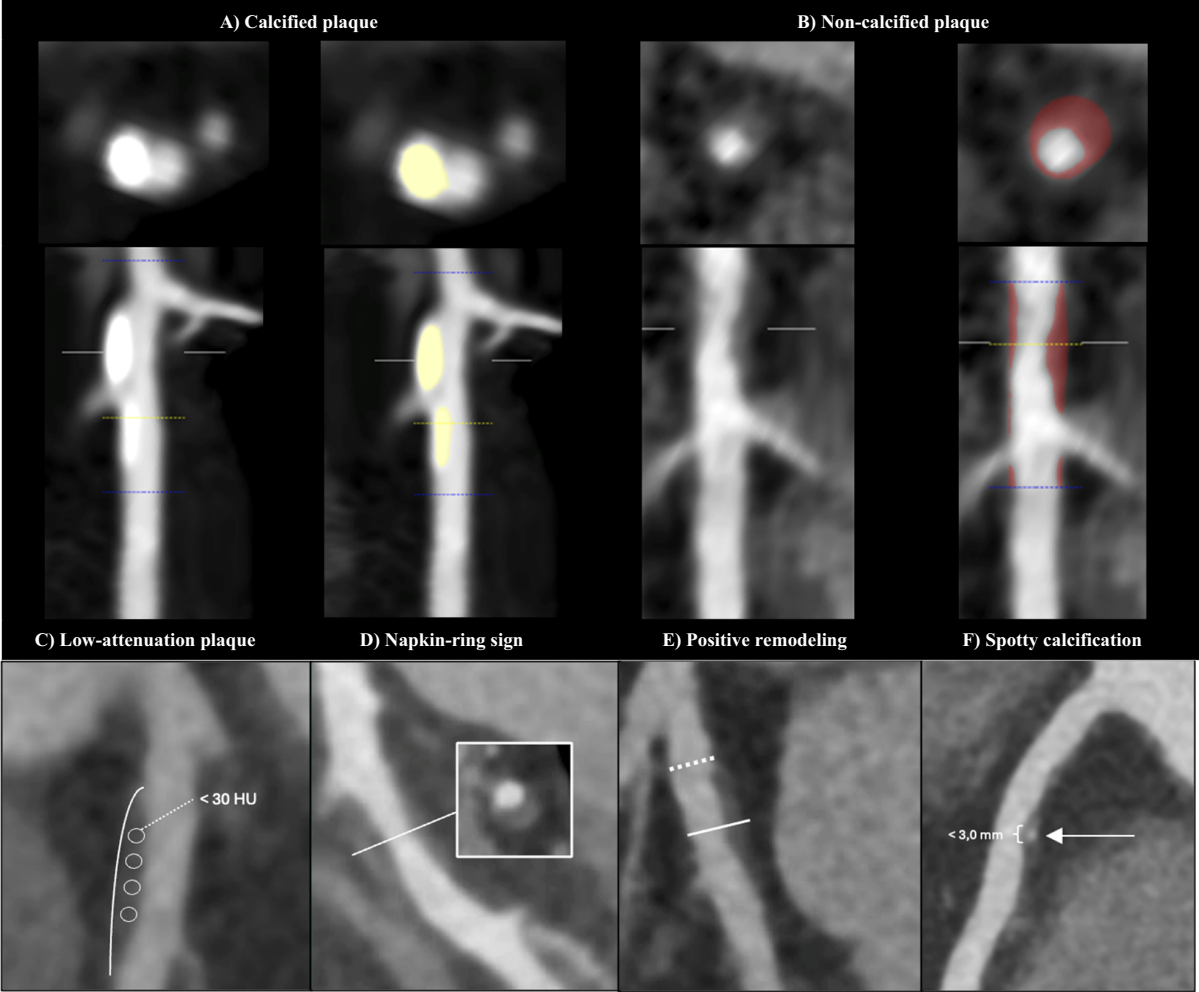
Coronary CT-derived plaque characteristics. Quantification of CP (A, yellow) and non-calcified (B, red) in cross-sectional and longitudinal view using a semi-automated plaque analysis software (AutoPlaque v.2.9, Cedars-Sinai Medical Center). C LAP (plaque with attenuation < 30 HU). D NRS (plaque with a core of low CT attenuation surrounded by a rim-like area of higher attenuation). E PR (ratio of vessel diameter at plaque site to the diameter of reference segment proximal to the lesion of ≥ 1.1). F SC (lesion containing calcium deposit < 3 mm within an arc of < 90° of cross-sectional images)
